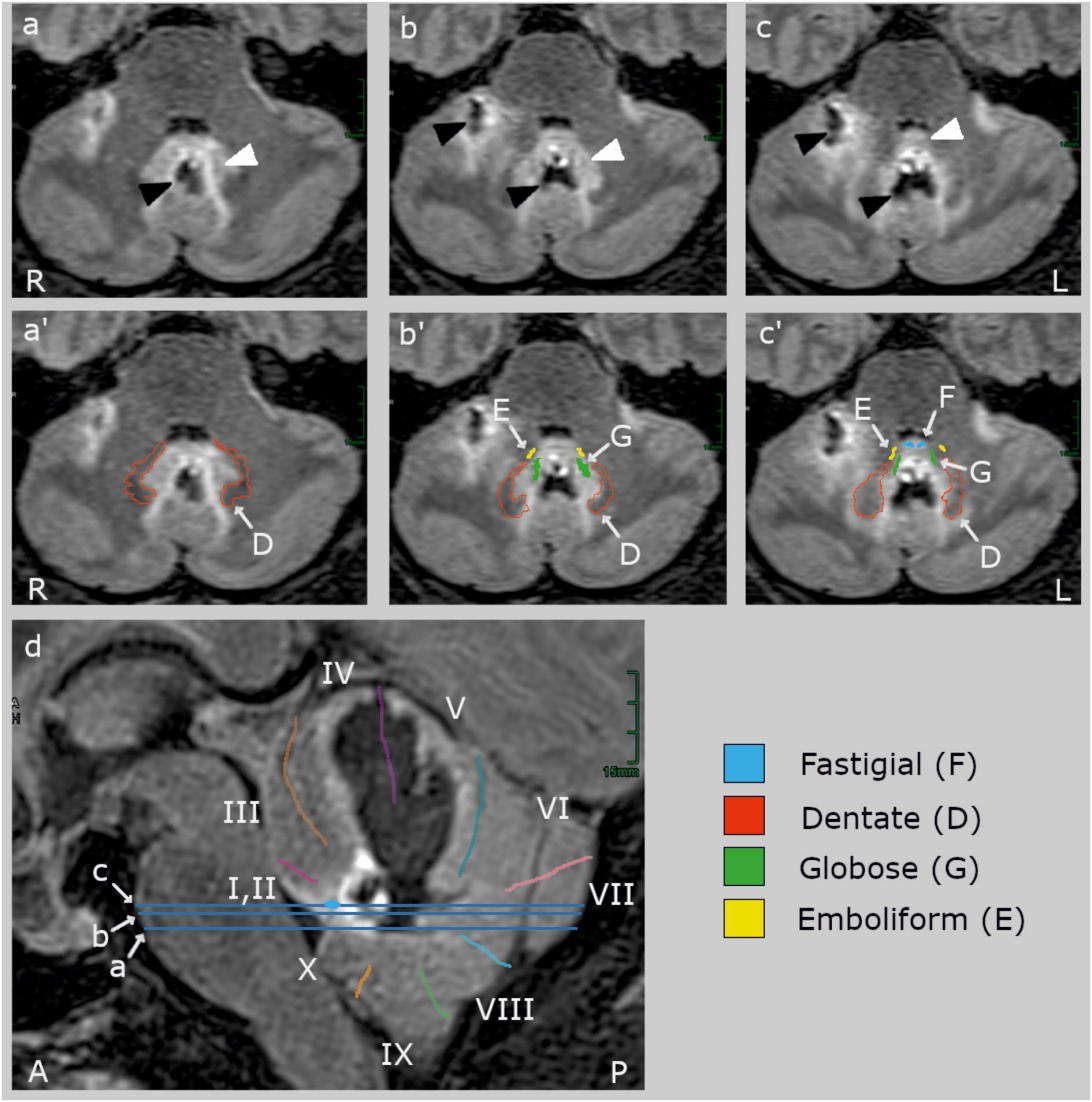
Clinical high-resolution FLAIR images (Siemens Vida 3 T MRI, Erlangen, Germany) with axial (a–c) and sagittal slices (d). The blue lines on the sagittal slice indicate the location of the axial slices. The upper row (a–c) shows the lesion (black arrowheads) and its edema (white arrowheads) without cerebellar landmarks which are labeled in the middle (a'–c') and lower row (d). The hemorrhage (hypointense, black) centered in the midline between the deep cerebellar nuclei and extended rostrally into the vermal lobules IV and V and the right hemispheric lobules V and VI (40, 41). The edema (hyperintense, light gray) involved bilaterally the fastigial nucleus (F, blue) anteriorly to the hemorrhage at the roof of the fourth ventricle, most likely impairing inter-fastigial projections (15), and laterally the interpositus composed of the globose (G) and emboliform (E) nuclei, and the medial part of the dentate nucleus (D). The lesion did not comprise (oculomotor) hemispheric lobule VI (simple lobule), the posterior oculomotor vermal lobules VI and VI (OMV), flocculus, paraflocculus, and caudal vermal uvula and nodulus. There was no brainstem lesion.
